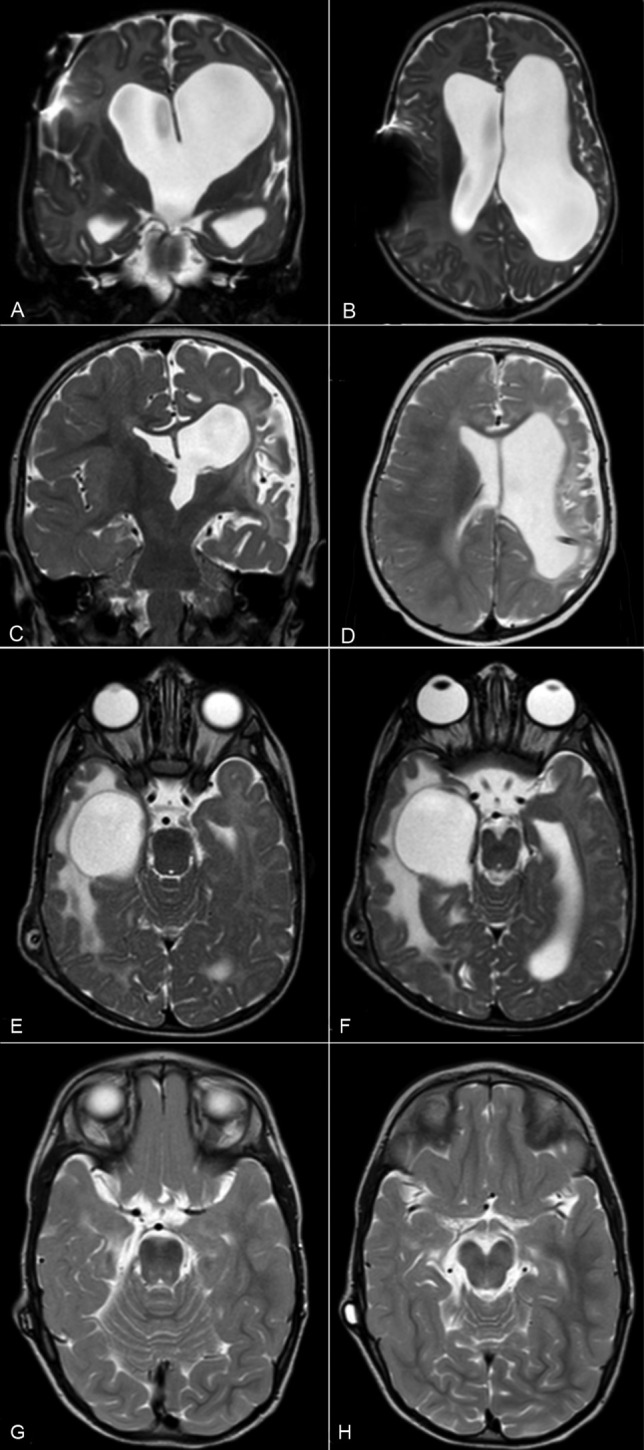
Preoperative coronal (A) and axial (B) T2-weighted MRI sequence and postoperative coronal (C) and axial (D) T2-weighted MRI sequences of a child suffering from unilateral ventricular enlargement. Preoperative axial (E, F) and postoperative axial (G, H) T2-weighted MRI sequence of a patient with entrapped temporal horn. In the postoperative images, the ventricular loculation is no longer visible
